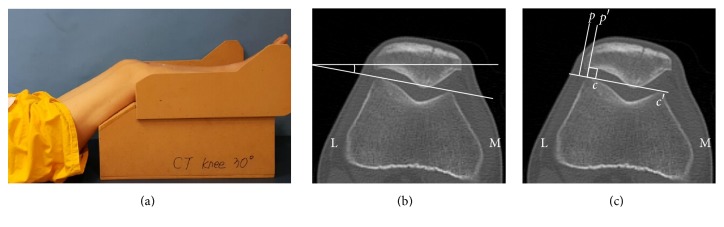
Methods of assessing lateral patellar tilt (LPT) and lateral patellar shift (LPS): (a) patient positioned for computed tomography imaging with knee in 30 degrees of flexion; (b) LPT, defined as the angle between a line intersecting the widest bony structure of the patella and a line tangential to the anterior femoral condylar surfaces; (c) LPS, defined as the ratio of distances *pp*′/*cc*′, where *cc*′ is the distance between the summits of the medial and lateral femoral condyles and *pp*′ is the distance between the summit of the lateral femoral condyle and the point where a line from the lateral edge of the patella perpendicular to the line that passed through the summits of the femoral condyles crosses that line. L, lateral; M, medial.
